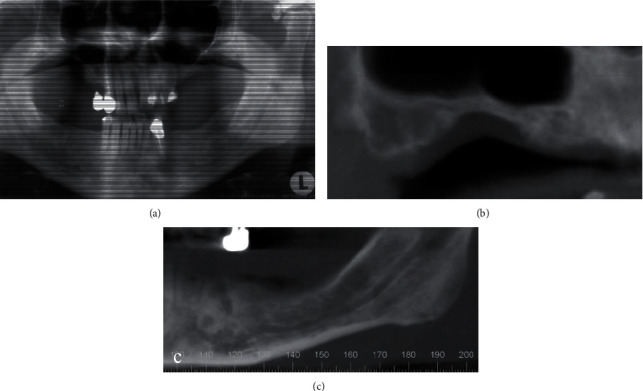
5-year follow-up radiographs: (a) panoramic radiograph; (b) sagittal CBCT image showing complete bone healing in the maxilla; (c) sagittal CBCT image showing complete bone healing in the mandible.
